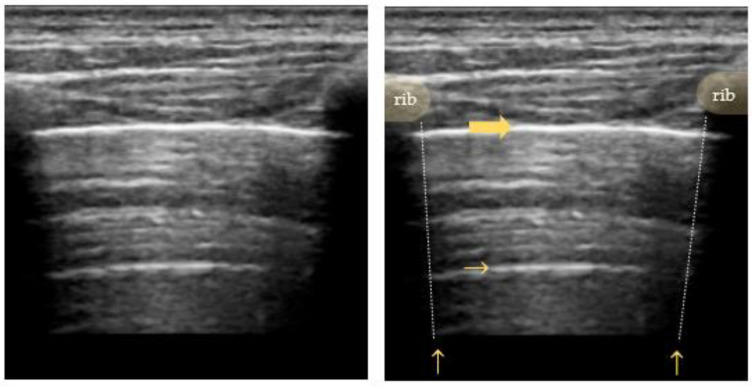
Bat sign: ribs and their sonographic shadow are outlined; the fat arrow points to the pleural line; the small horizontal arrow points to the A-line (reverberation artifact of the pleural line); the vertical arrow points to a rib’s shadow line.
